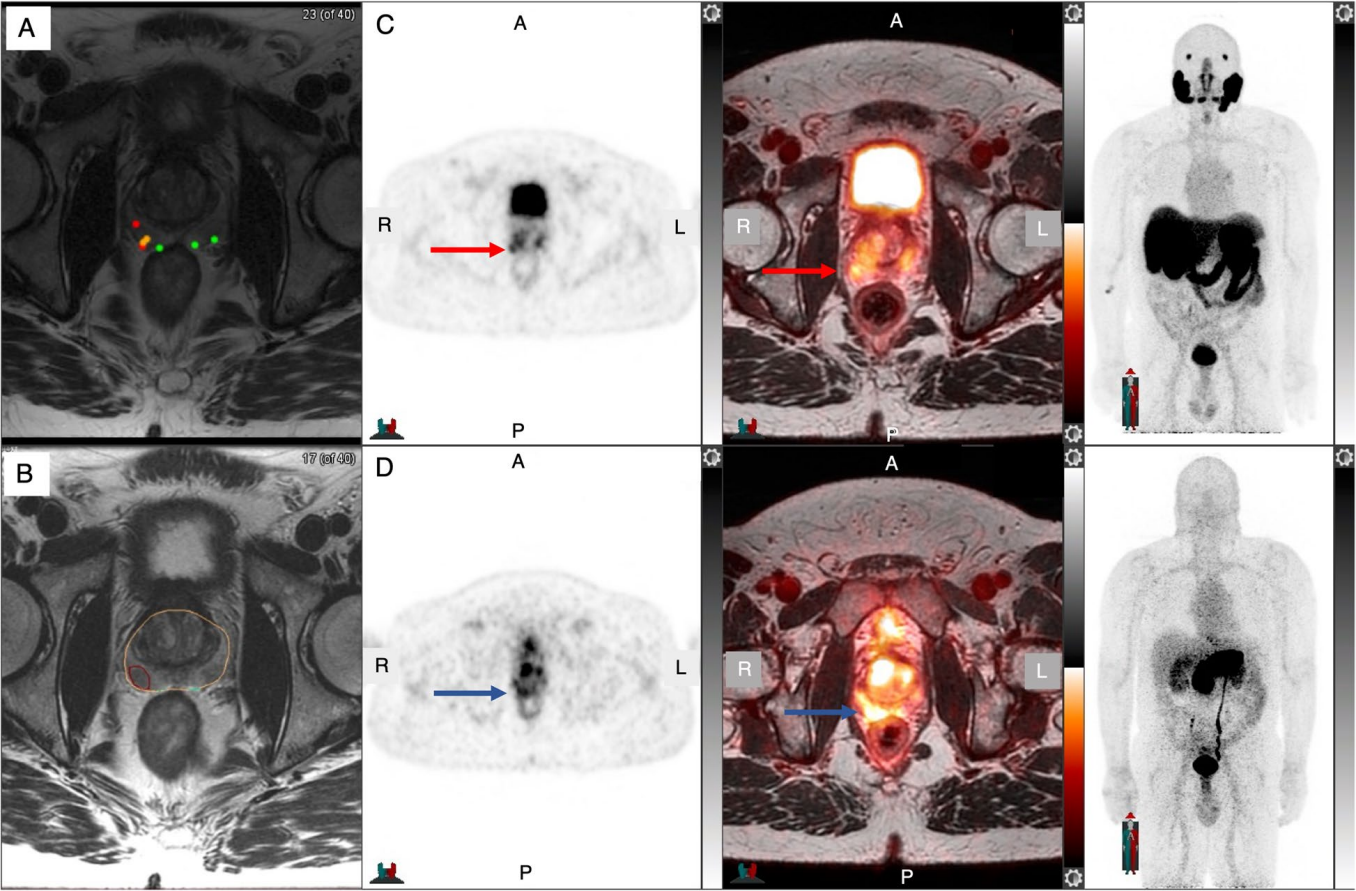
62-year-old man with PSA 7.0 ng/mL and PSA density 0.24 ng/mL^2^. MRI shows a PI-RADS 4 lesion in the right lateral base with color coded needle tracks from biopsy; green-benign, yellow-Gleason score 3 + 3, red-Gleason score 3 + 4 or higher A, and target tumor volume B. ^68^ Ga-PSMA11 C and ^68^ Ga-RM2 D axial PET, axial fused PET/MRI, and MIP show congruent focal uptake in the right prostate lesion. The lesion was treated with HIFU; resolution on both ^68^ Ga-PSMA11 and ^68^ Ga-RM2 PET/MRI was seen 6 months after treatment
